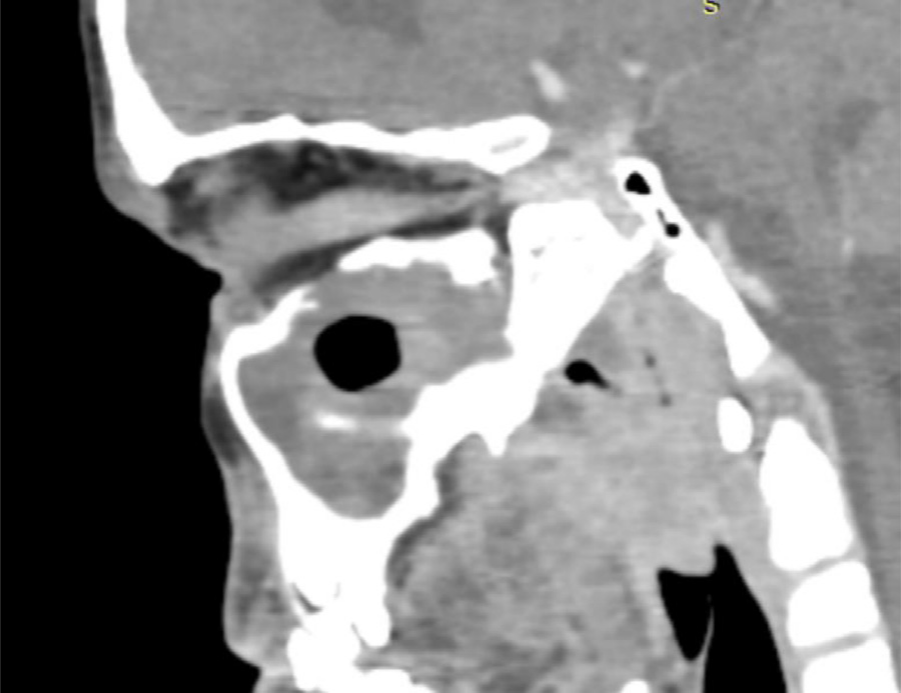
The facial CT sagittal section shows a heterogeneous tissue lesion centered on the left maxillary sinus with lysis of the upper wall and invasion of extraconal fat.
